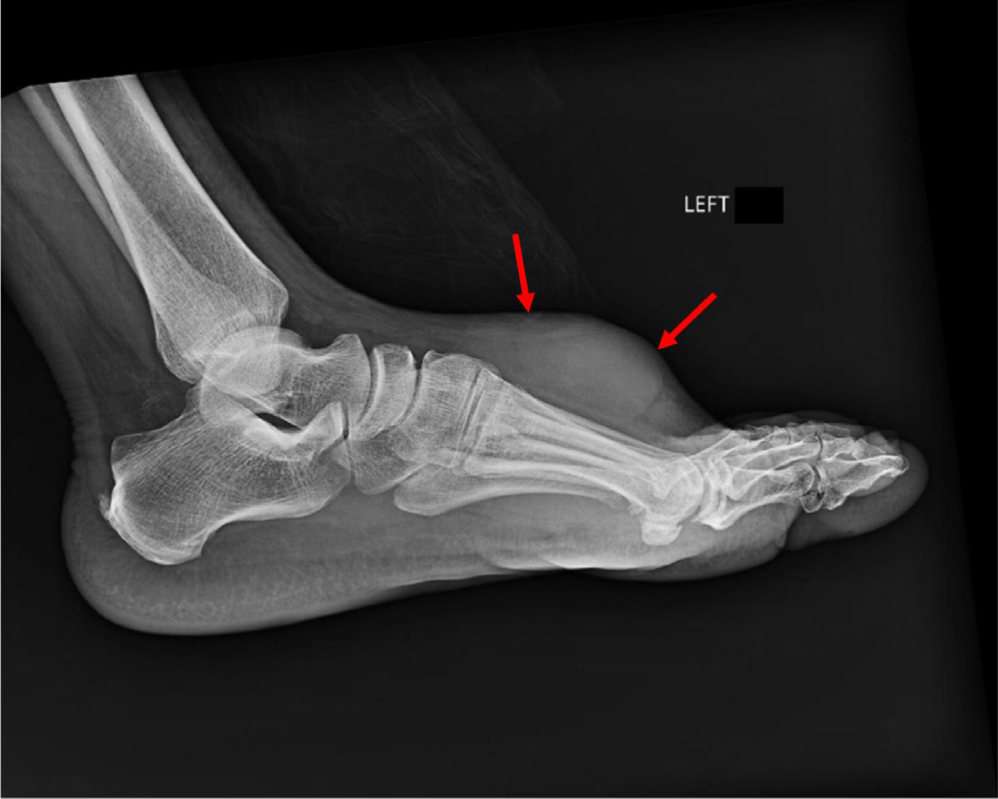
Radiograph of the left foot in a lateral projection demonstrating a large soft tissue mass over the dorsum of the foot (arrows). There is no evidence of bony erosion or fractures.
